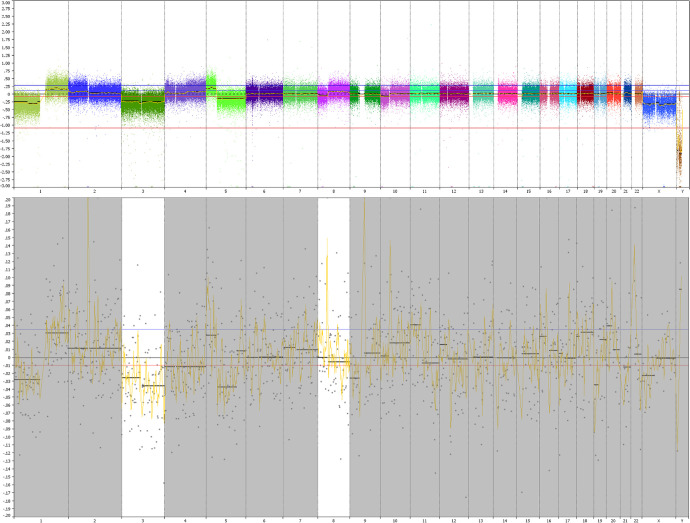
Corresponding CNV-profiles in patient harboring metastases. This patient developed several diffuse metastatic lesions in the liver, spleen, and spine. (A) Copy number variations of the primary tumor were acquired by single nucleotide polymorphism array of tumor tissue obtained after enucleation. The primary tumor shows a loss of chromosomes 1p, 3, 5q, and 10p; and a gain of chromosomes 1q and 8q. Tumor tissue is processed by the pathology laboratory within 4 hours after removal of tissue and subsequently molecular analyses is performed. In addition to routine diagnostics, peripheral blood was obtained after detection of metastases ultrasound of the liver that was later confirmed by computed tomography imaging. After in silico size selection, the loss of chromosomes 1p, 3, 8, and 10 and the gain of chromosome 1q were confirmed in the blood of this patient.
